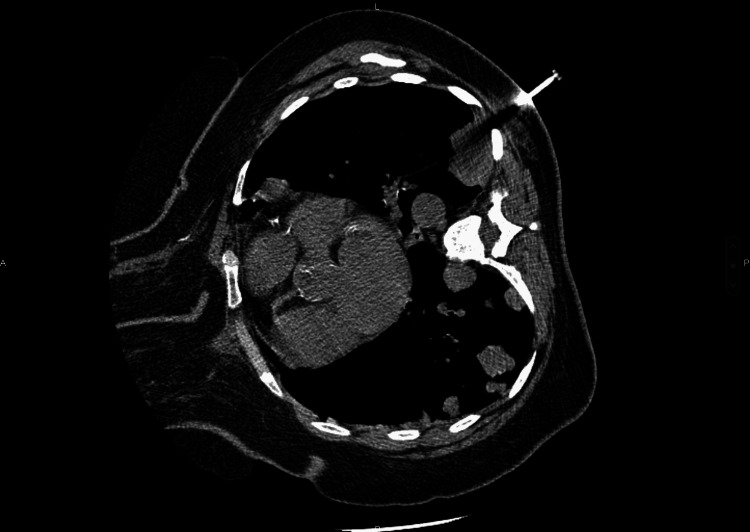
CT-scan guided trans parietal biopsy of a lung mass, revealed to be a lung large cell neuroendocrine carcinoma on pathological assessment
